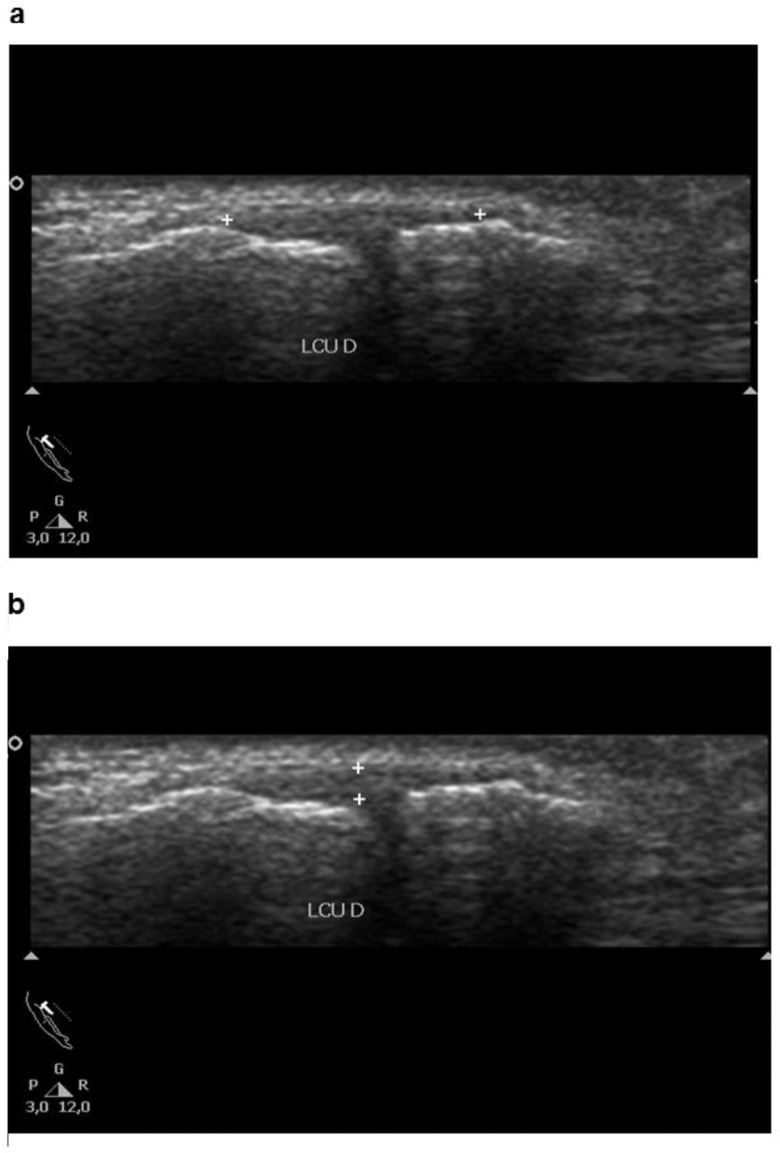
Ultrasonographic images showing the length (a) and thickness (b) of the collateral ulnar ligament of the thumb.
